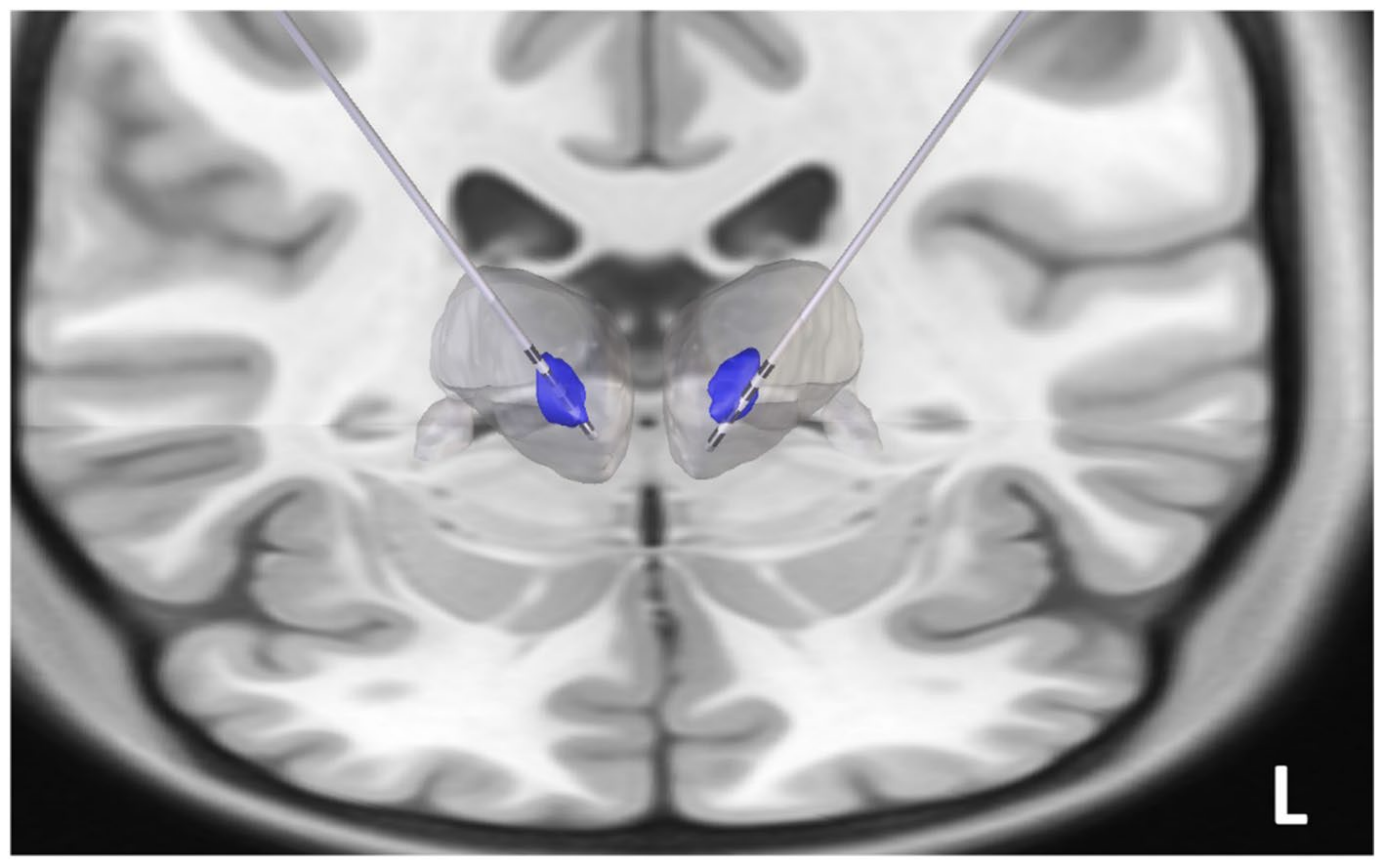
Reconstruction of the centromedian (CM) thalamic deep brain stimulation (DBS) electrodes: The post-implant CT scan was coregistered to pre-operative 3 T FGATIR images (Fast Gray Matter Acquisition T1 Inversion Recovery). Thalamus Optimized Multi Atlas Segmentation (THOMAS) segmentation algorithm was used to generate patient specific thalamic nucleic segmentation (blue regions represent the bilateral CM nuclei, the gray blob represents the thalamic boundary). DBS electrodes (Medtronic 3,389) were noted to be implanted in the CM thalamus.
